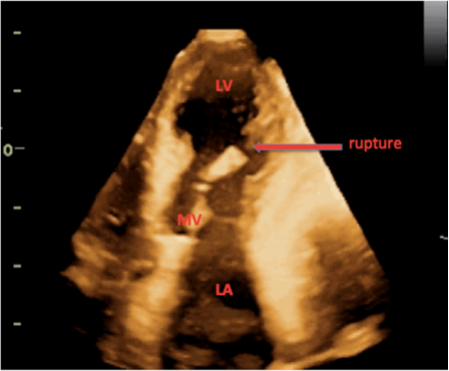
Ruptured mitral papillary muscle post infarction seen by 3D echocardiography from the apical four-chamber view view. *LA* left atrium, *LV* left ventricle, *MV* mitral valve
